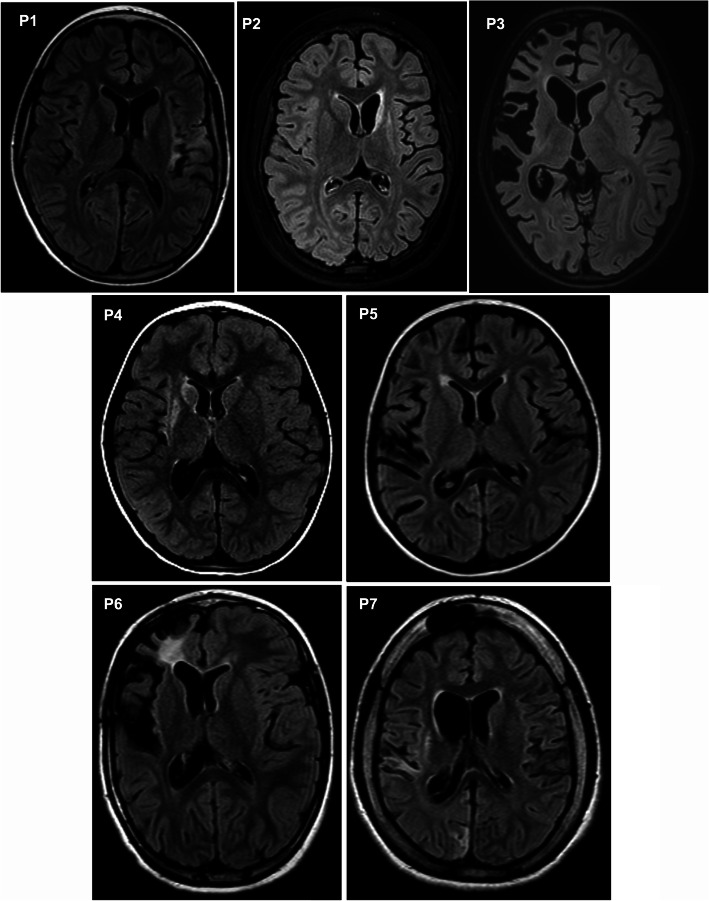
Magnetic resonance imaging (MRI) at the time of surgery. Axial fluid-attenuated inversion recovery (FLAIR) MRI sequences of the brain showing differently pronounced atrophy of the affected hemisphere in every patient at the time of surgery are displayed. In addition, various inflammatory and glial changes in the affected hemisphere can be observed in every patient
